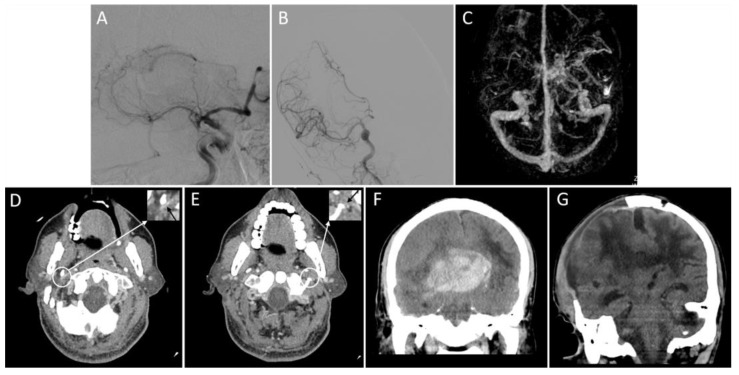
Representative images of the outflow profile of internal jugular veins and outcomes. An adult patient with an acute right middle cerebral artery occlusion (A) was admitted with a National Institutes of Health Stroke Scale score of 19. The patient received a thrombectomy for 6 h following stroke onset and achieved successful reperfusion (modified thrombolysis in cerebral infarction score = 3) (B). Three-dimensional reconstructed computed tomography (CT) venography shows that the right transverse sinus is larger than the left (C), and bilateral internal jugular veins are severely narrowed on the axial CT angiography (D,E, black arrows). On the non-contrast CT scan within 24 h after thrombectomy, a parenchymal hematoma, midline shift, and subfalcine hernia are present (F). Sixteen days after thrombectomy (15 days after decompressive craniectomy), there is an apparent cerebral edema (G). The patient’s modified Rankin Scale score at discharge is 5.
